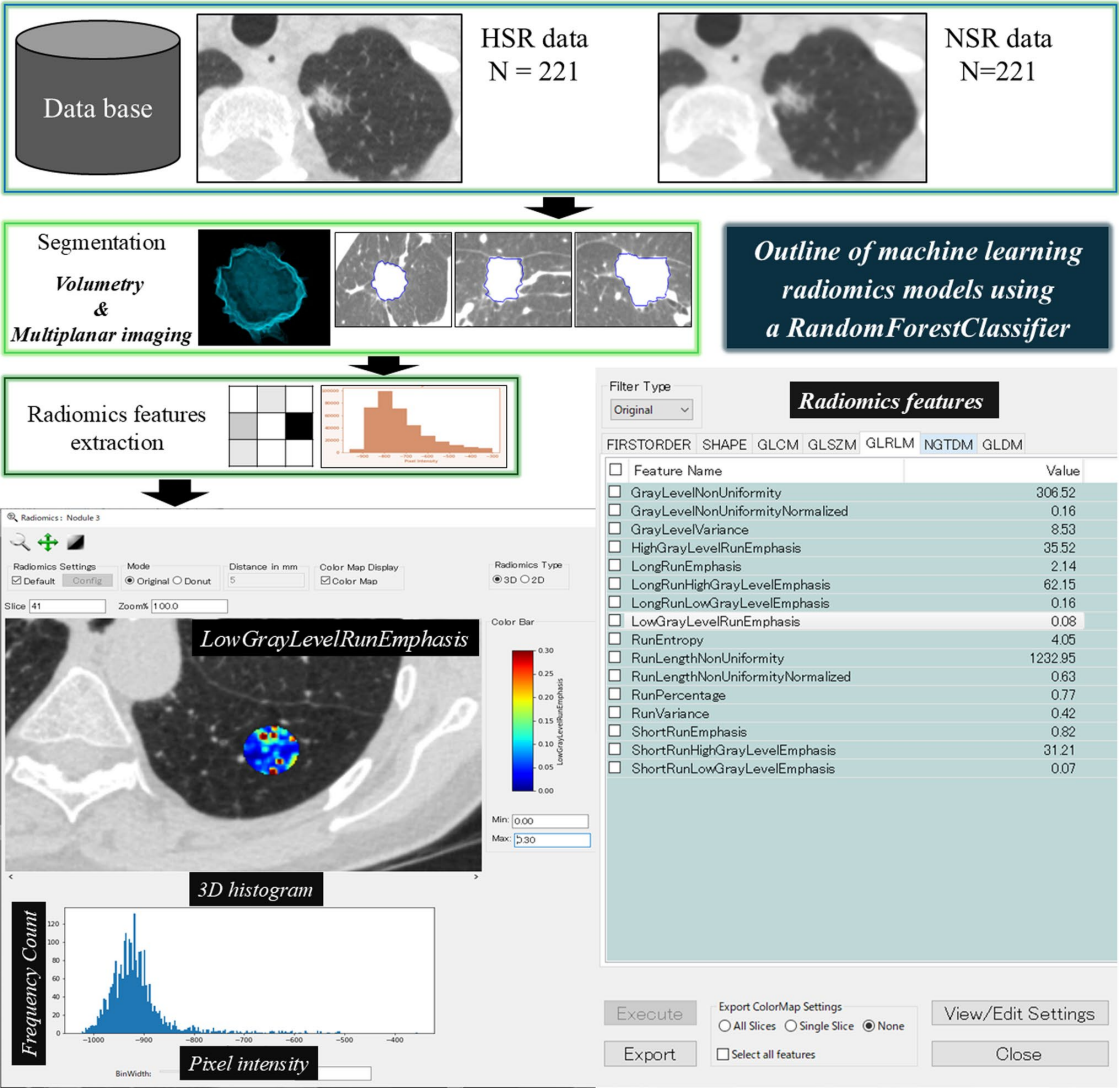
Outline of machine learning radiomics models using a RandomForestClassifier in training cohort. Nodules are automatically extracted from any CT image data, and analysis results are output using 3D CT histograms and color map displays of radiomics features. *HSR* high-spatial-resolution, *NSR* normal-spatial-resolution
